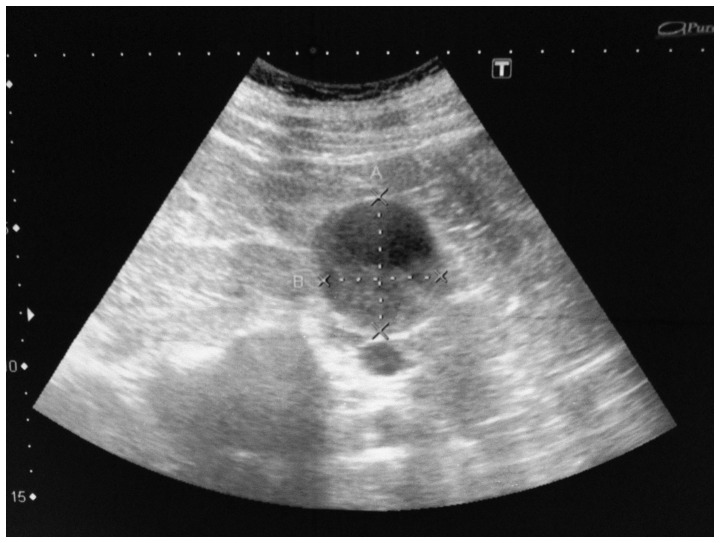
Ultrasonography of the cyst prior to surgery.
